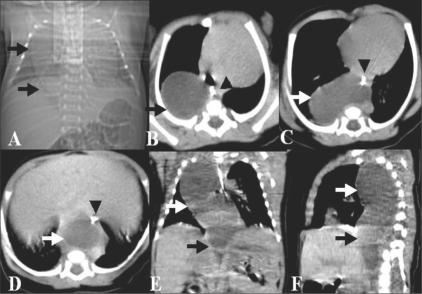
Postnatal CT scan: Scannogram (A) shows a right mediastinal opacity (arrows). Axial (B–D), coronal (E), and oblique sagittal reformatted (F) sections show the posterior mediastinal thoracoabdominal cyst (arrows). A paraesophageal course is seen. The esophagus is delineated by the feeding tube (arrowheads)
